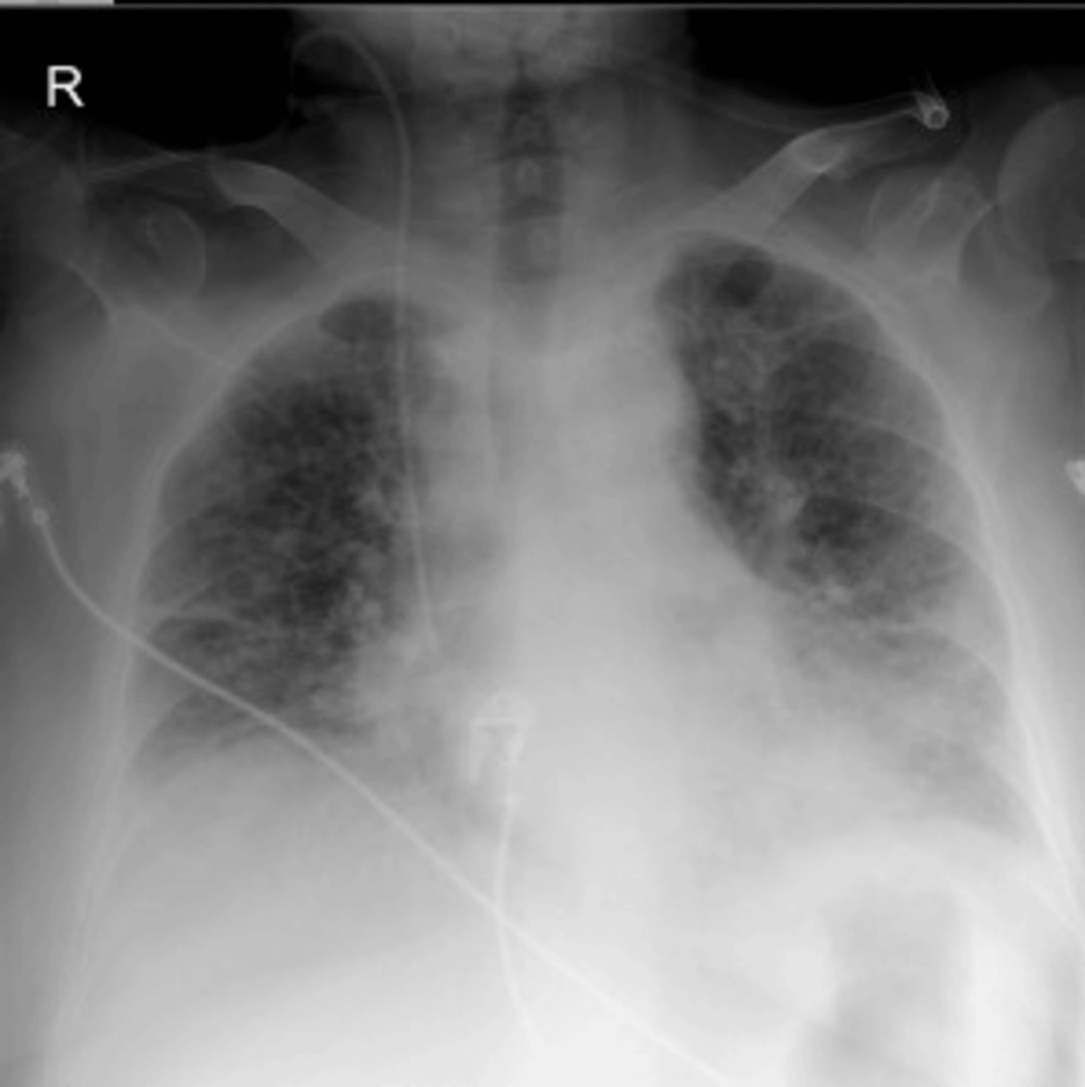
Follow-up chest radiograph obtained at this time demonstrating interval improvement in pulmonary infiltrates
